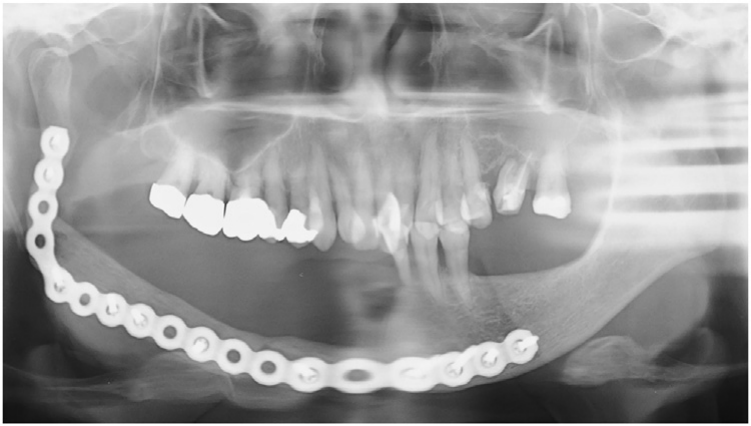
Orthopantomography at 1 year postoperatively. The occlusion between the residual mandibular teeth and the maxillary teeth is optimal.
